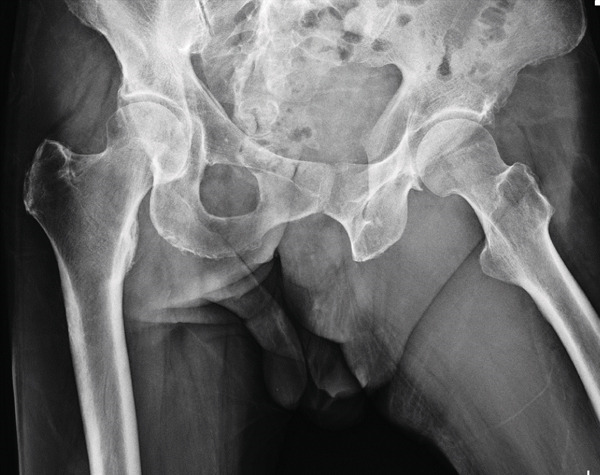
Case 1. Preoperative anteroposterior radiographs of bilateral hip joints.
